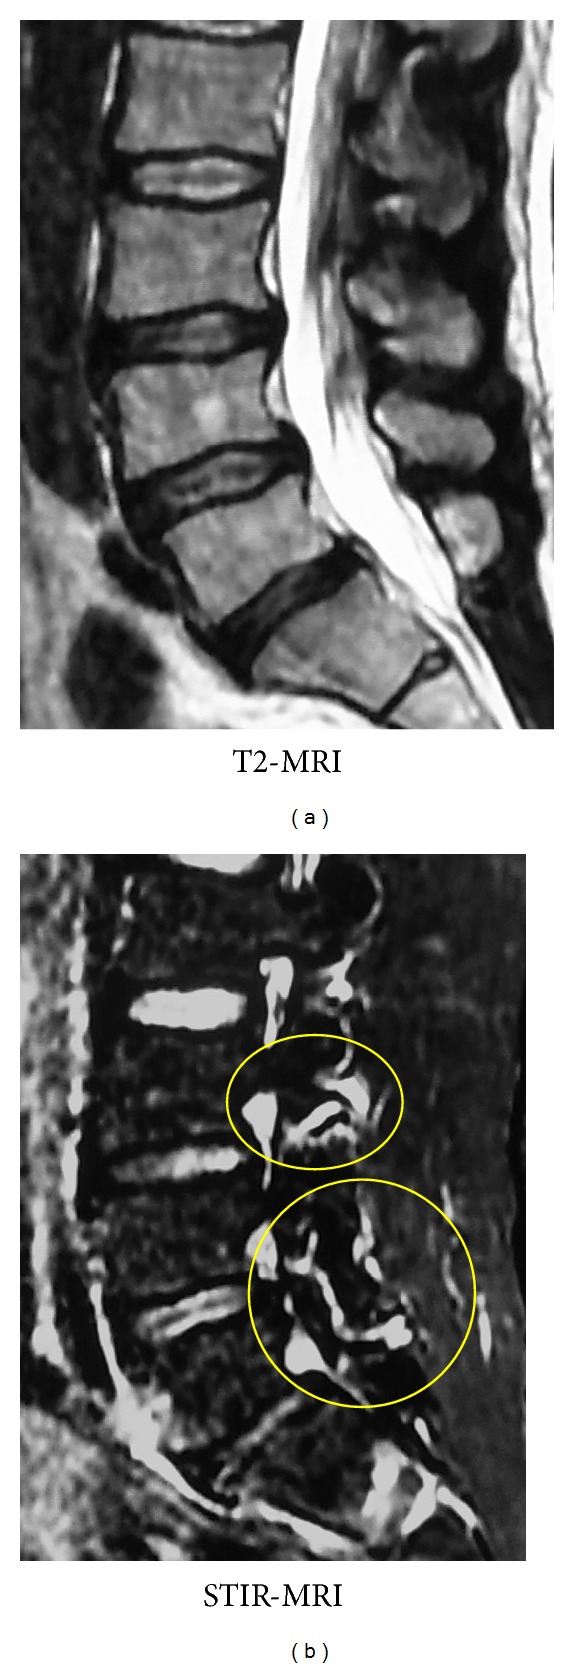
MRI at initial presentation shows in the left panel no obvious degenerative spinal disorder such as herniated nucleus pulposus or spinal canal stenosis. Slight disc degeneration at L3-L4, L4-L5, and L5-S1 is evident. In the right panel, effusion is also seen around the pars defects and surrounding facet joint.
